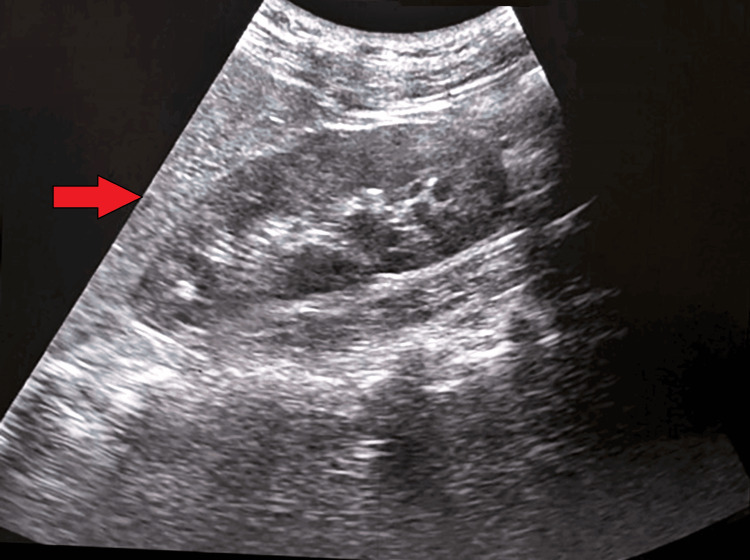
Ultrasonography of the kidneys, ureters, and bladder (USG-KUB) suggested grade 1 renal parenchymal disease (arrow)
